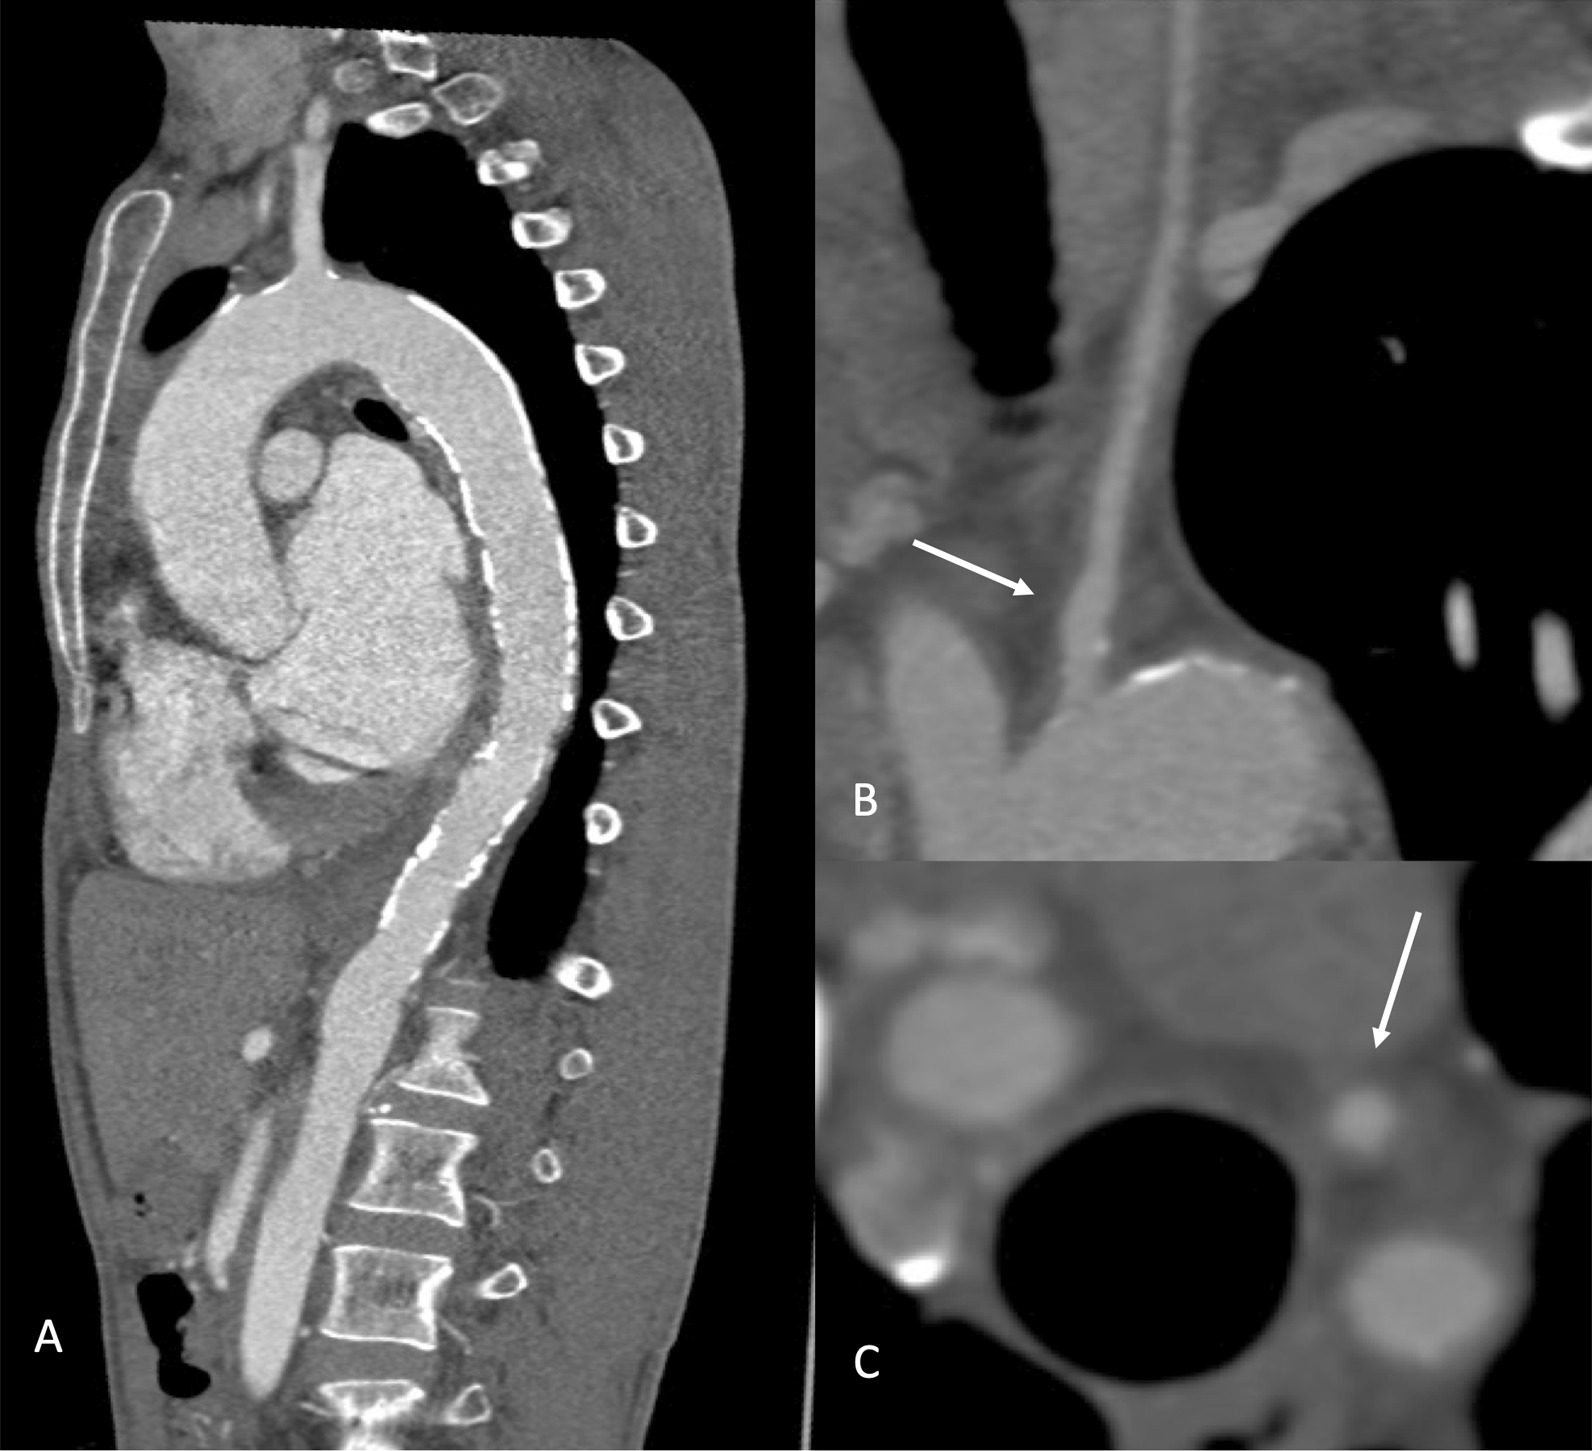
Computed tomography angiography of the aorta. Mural calcification extended from the arcus toward the abdominal aorta, characterizing the pathognomonic sign of Takayasu arteritis (TA) (a). An active lesion of TA was spotted in the left common carotid artery. The arrows pointed to a noncalcified mural thickening in longitudinal (b) and perpendicular (c) views
